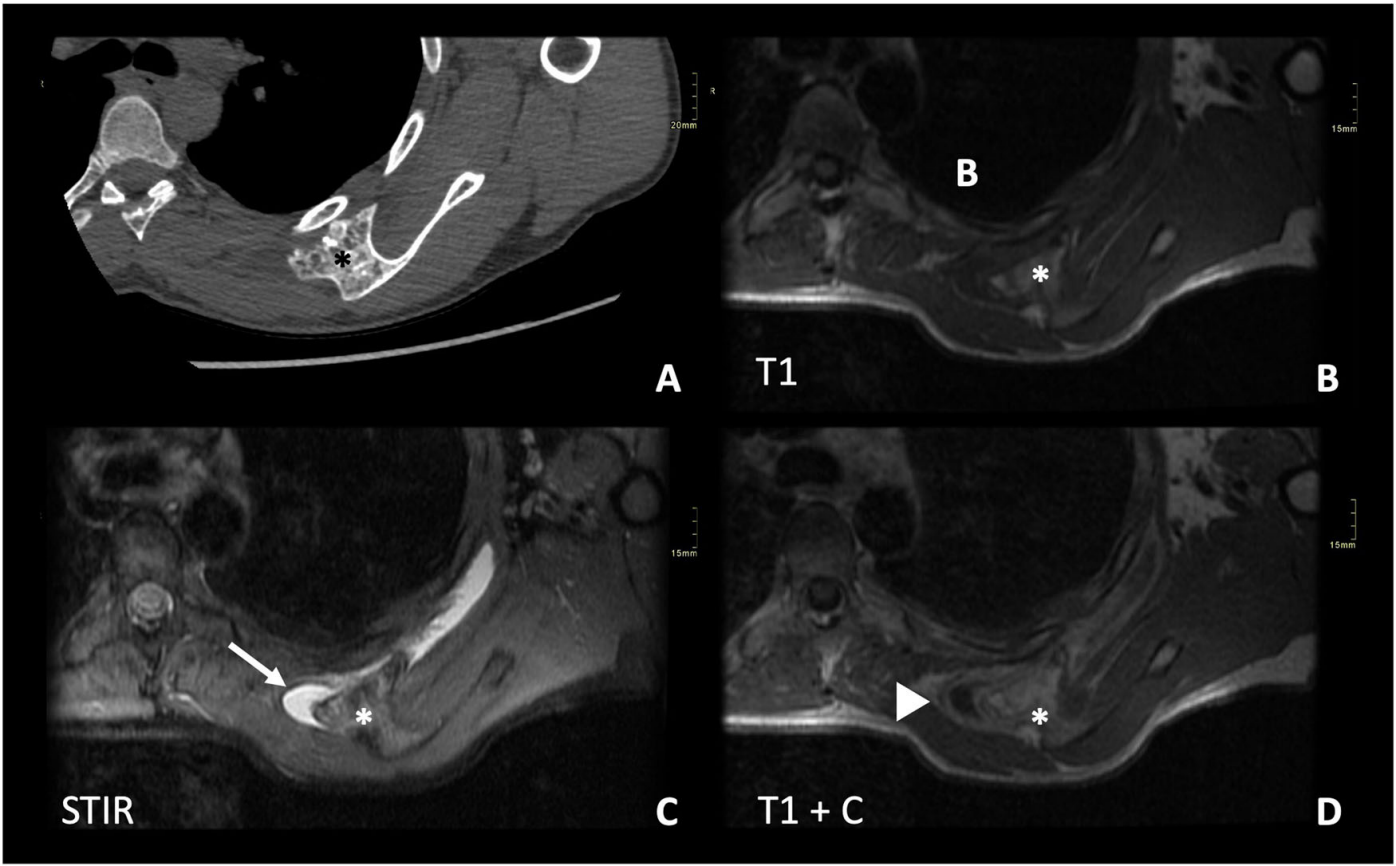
A 28-year-old male diagnosed with HME presents with pain in his left shoulder. Axial CT scan (A) and MRI with axial T1W (B), STIR (C), and T1W post-contrast T1 (D) sequences. There is an osteochondroma (*) in the scapula, showing key features such as cortical and medullary continuity with the underlying bone. There is a soft tissue mass surrounding it, with low SI in T1 and high SI in STIR, consistent with a bursa (arrow). The post-contrast sequence shows enhancement of the wall of the bursa (arrowhead), which suggests inflammation
